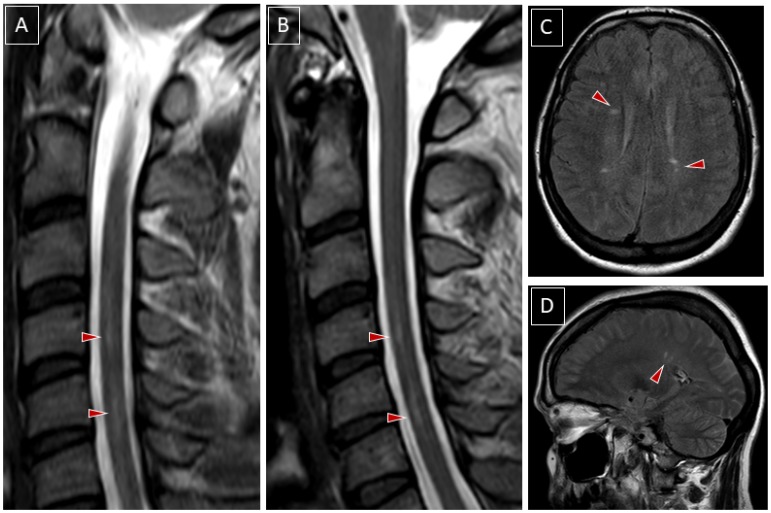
Radiological features of longitudinally extensive myelitis related to the tick-borne encephalitis virus (TBEV) which caused a Polio-like illness. Panel (A). Spinal cord MRI T2 sagittal images in the acute phase. The presence of a tractopathy involving the central and anterior section of the cervical spinal cord which expands over more than three vertebral bodies is depicted by red arrows. Panel (B). A follow-up scan one month later demonstrates a slight reduction of the hyperintense signal, shown by the red arrows. Panels (C,D). Fluid-attenuated Inversion recovery (FLAIR) MRI (axial and sagittal planes) revealed several oval-shaped hyperintensities perpendicular to the ventricle system, consistent with white-matter disease, shown by the red arrows.
